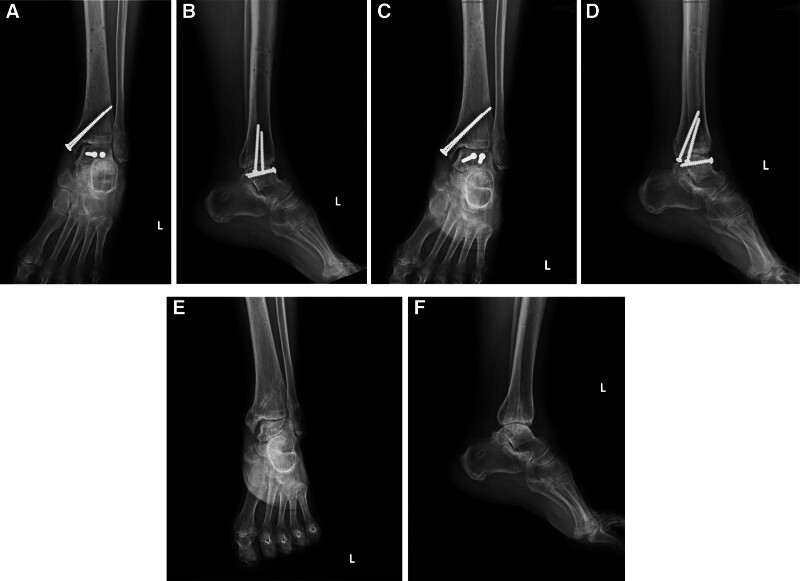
AP (A) and lateral (B) radiographs obtained 3 months after surgery demonstrating callus formation; radiographs showed bony union at 6 months after surgery (C, D); and after implant removal at 2 years after surgery (E, F).
